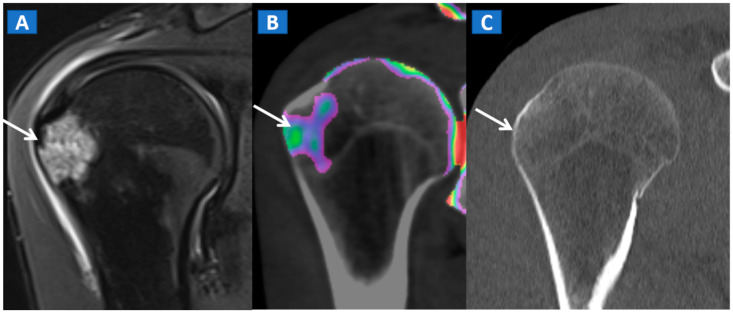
A 35-year-old male with traumatic BME of the humeral head. On the coronal STIR MRI image (A), post-traumatic BME of the humeral head is depicted as a hyperintense signal in the subcortical area (arrow). The presence of BME is confirmed on the 2D DECT coronal 1 mm reconstructed image (B) (arrow). The high resolution 1 mm coronal CT image with bone window (C), rules out the presence of cortical fractures.
